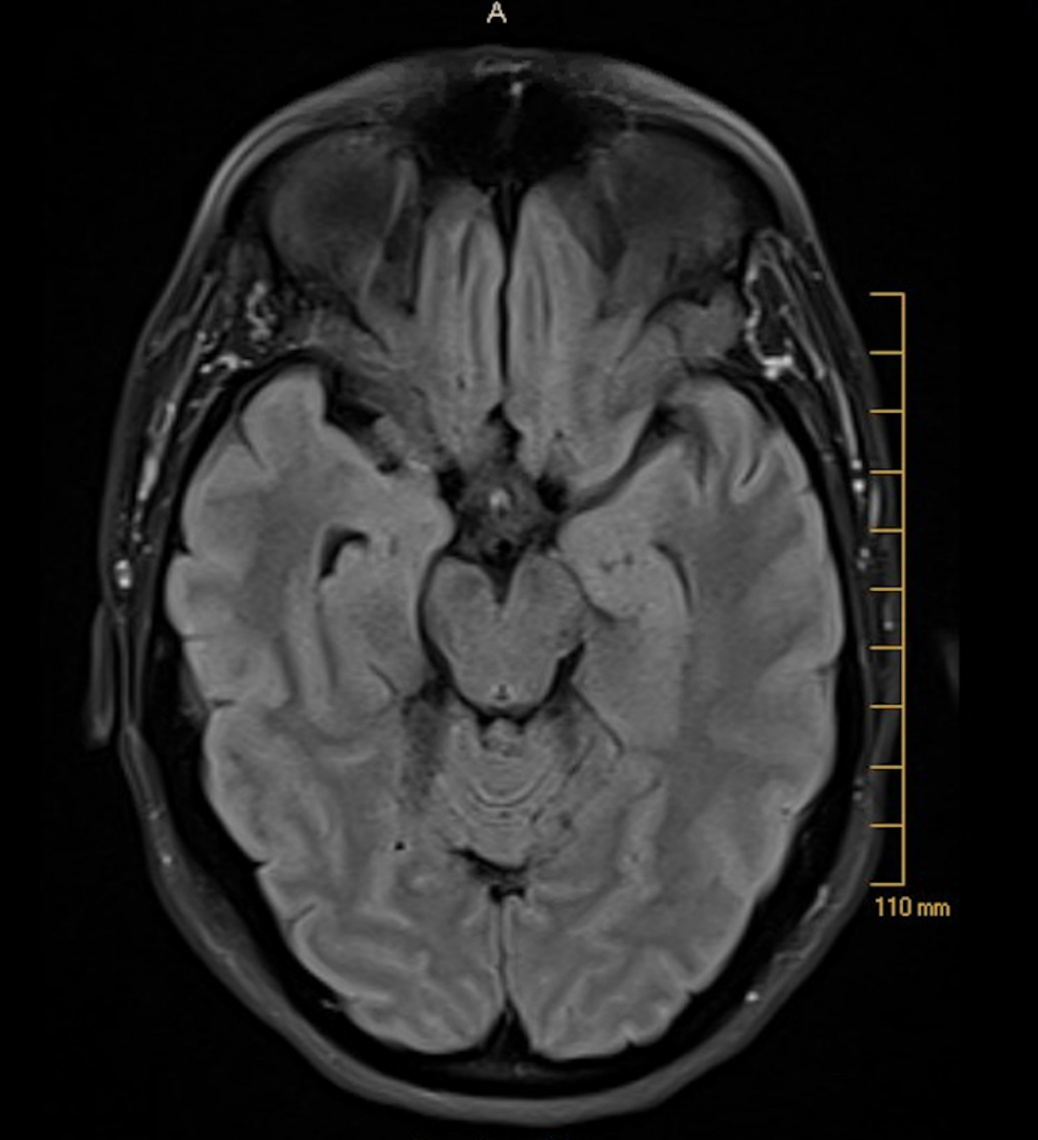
T2/FLAIR image of the axial section of the MRI brain at the level of the Sylvian fissure showing normal findings FLAIR: Fluid-attenuated inversion recovery; MRI: Magnetic resonance imaging.
